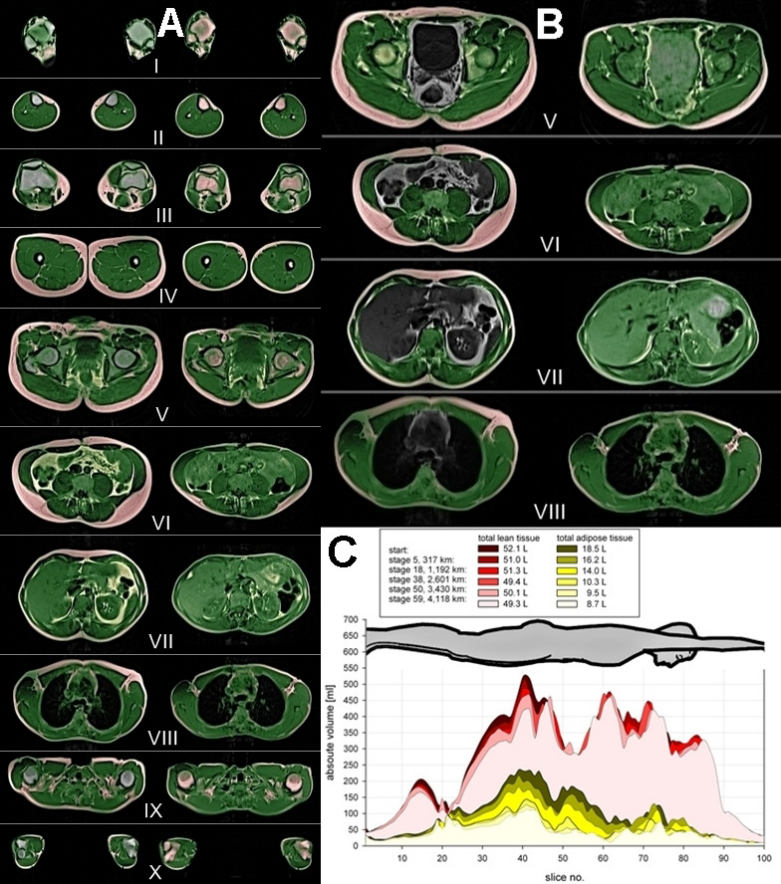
Semiautomatic tissue separation with mobile whole body MRI of a 32-year-old male finisher of the TEFR09. A: Right row (before start): green: total lean tissue, red: somatic adipose soft tissue, yellow: visceral adipose tissue, blue: adipose bone marrow. Left row (after 4,120 km): green: total lean tissue, red: somatic adipose tissue (= somatic adipose soft tissue + adipose bone marrow), yellow: visceral adipose tissue. (selected slices: I: ankles, II: middle of lower legs, III: knees, IV: middle of upper legs, V: hip/pelvis, VI: umbilical level, VII: upper abdomen, VIII: heart/mediastinum, IX: shoulder girth, X: elbows). B: Right row (before start): green: somatic lean tissue, red: somatic adipose tissue, grey: total visceral volume. Left row (after 4,120 km): green: total lean tissue, red: somatic adipose tissue (= somatic adipose soft tissue + adipose bone marrow), yellow: visceral adipose tissue, blue: intraluminal nutrition fat in intestinal tract. (selected slices: V: hip/pelvis, VI: umbilical level, VII: upper abdomen, VIII: heart/mediastinum). C: Loss of total lean and total adipose tissue during the TEFR09.
